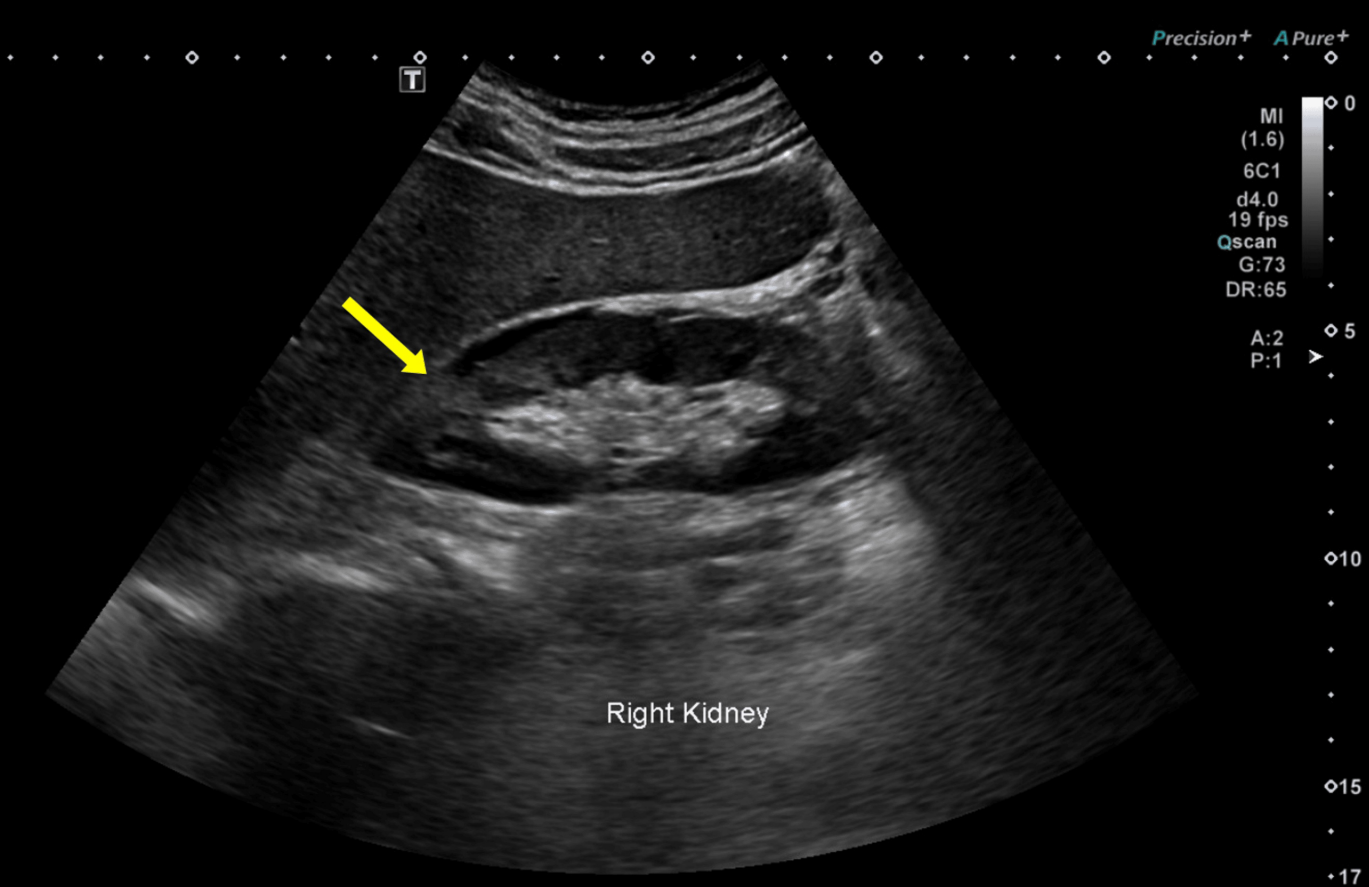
Initial ultrasound showing a hyperechoic area at the upper pole of the right kidney (yellow arrow) with mild perinephric fluid, suggestive of acute pyelonephritis.
